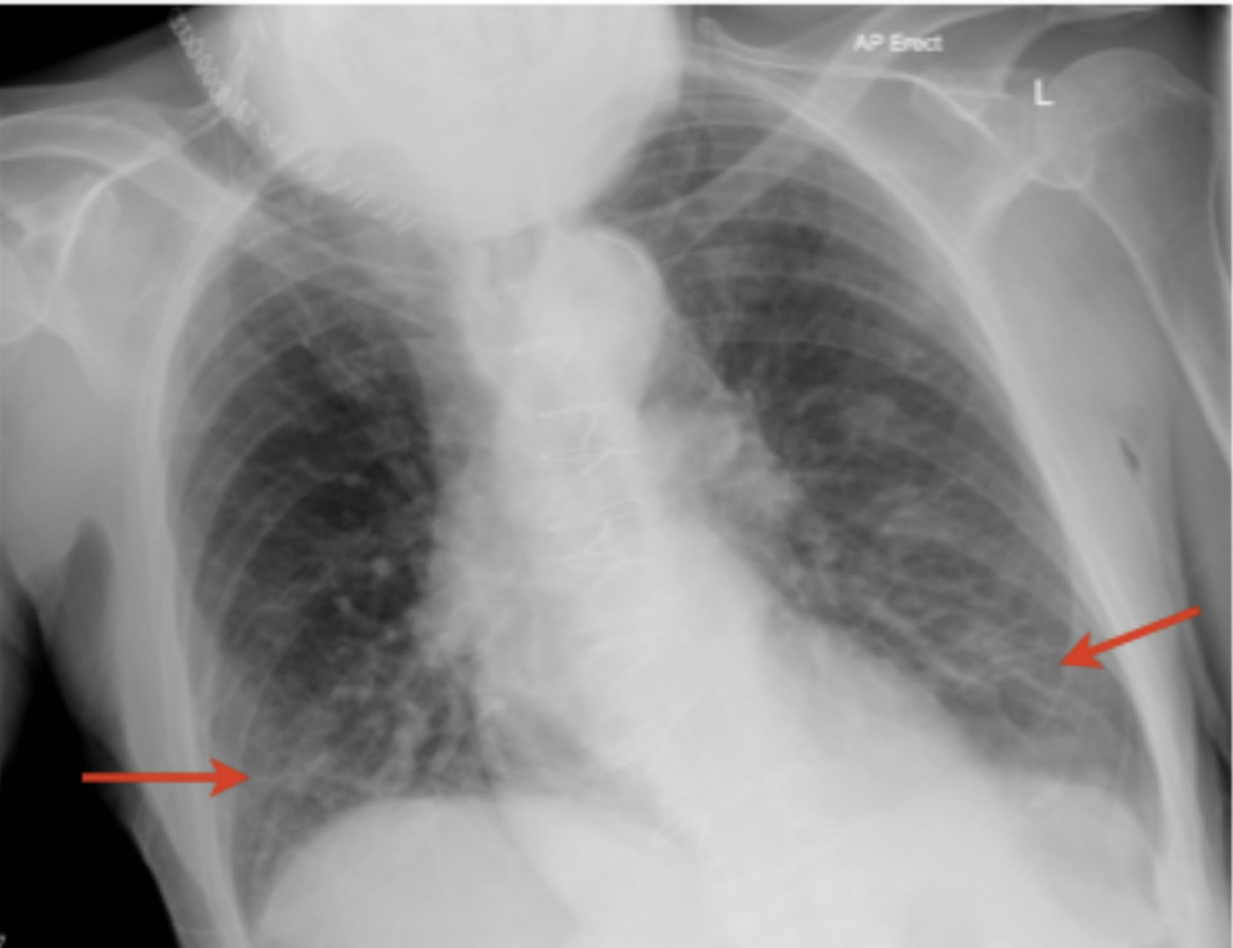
X-ray of chest. Scan taken 16 days post-admission, reported as having some minor airspace shadowing in both lower zones, which may be due to an infection.
